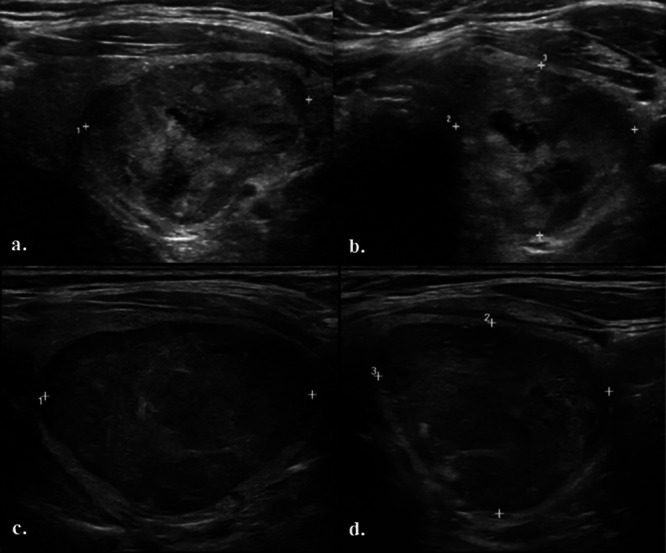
Thyroid ultrasonography. Previous ultrasonography from Spring 2015: a Sagittal and (b) axial images revealed 3.4 × 2.7 × 2.6 cm heterogeneous solid nodule with small cystic components in left thyroid gland. New ultrasonography from September 2023: c Sagittal and (d) axial images demonstrated mild increase in size of the nodule, now diffusely solid and hypoechoic measuring 4 × 3 × 3.5 cm.
